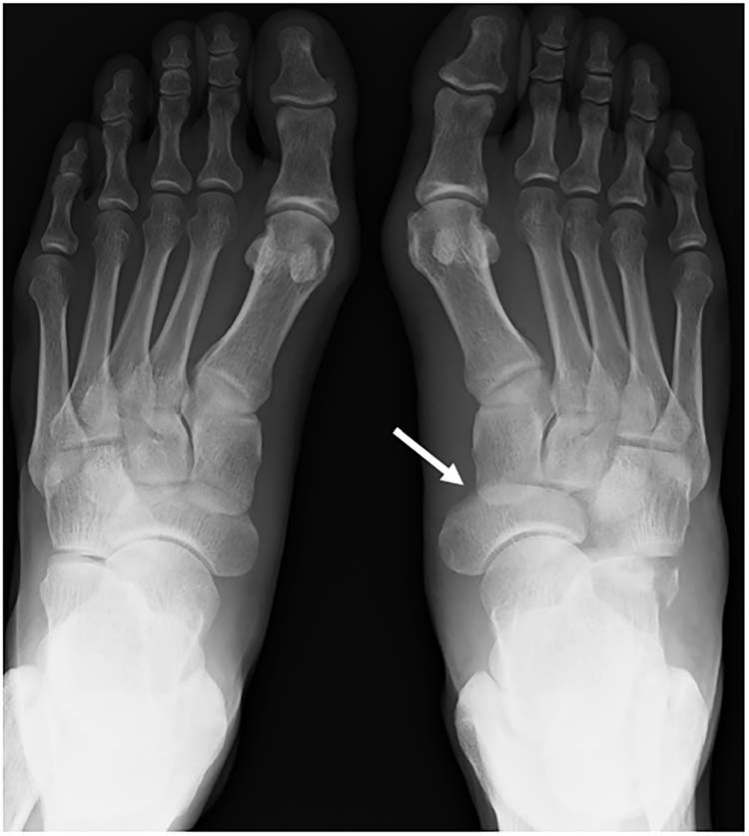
A plain radiograph shows lateral dislocation of the naviculocuneiform and calcaneocuboid joints of the right foot (arrow). The difference between the two sides is obvious.
